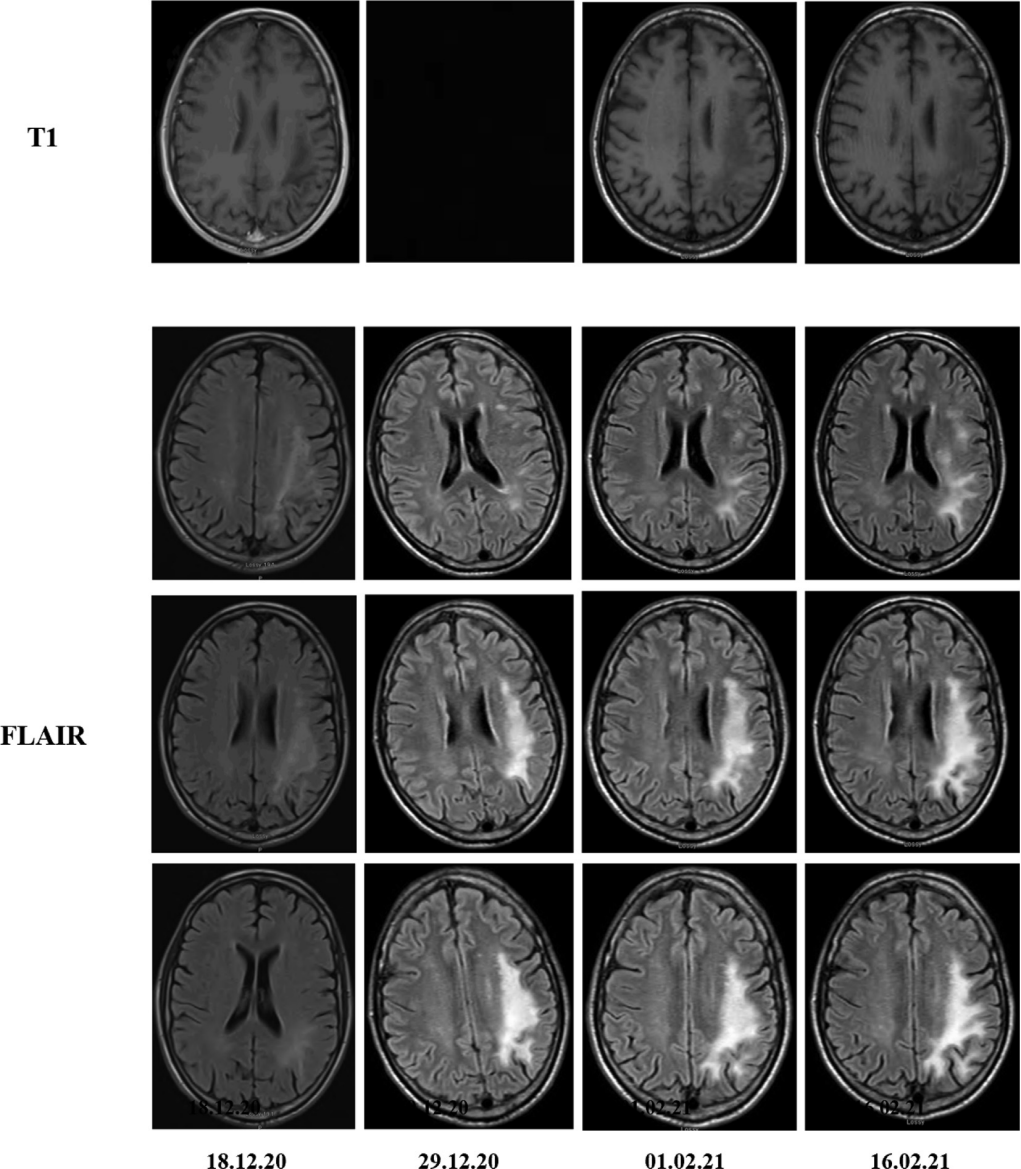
Progression of PML lesion over time on T1 and FLAIR imaging.
